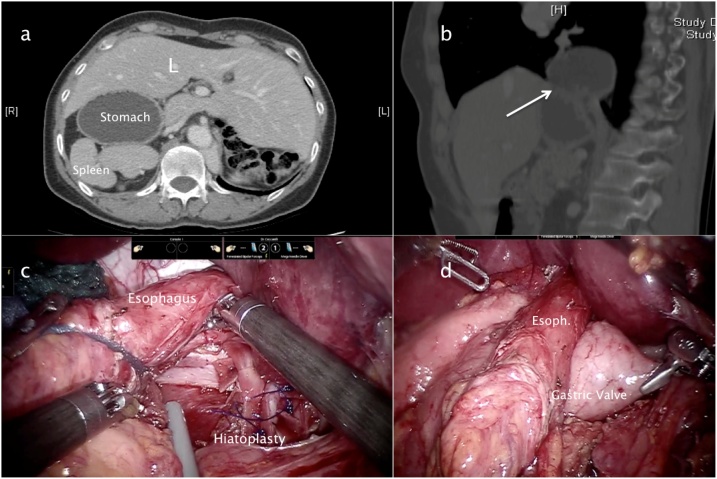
a–b) CT scan images, c) Posterior Hiatoplasty, d) Toupet fundoplication.
